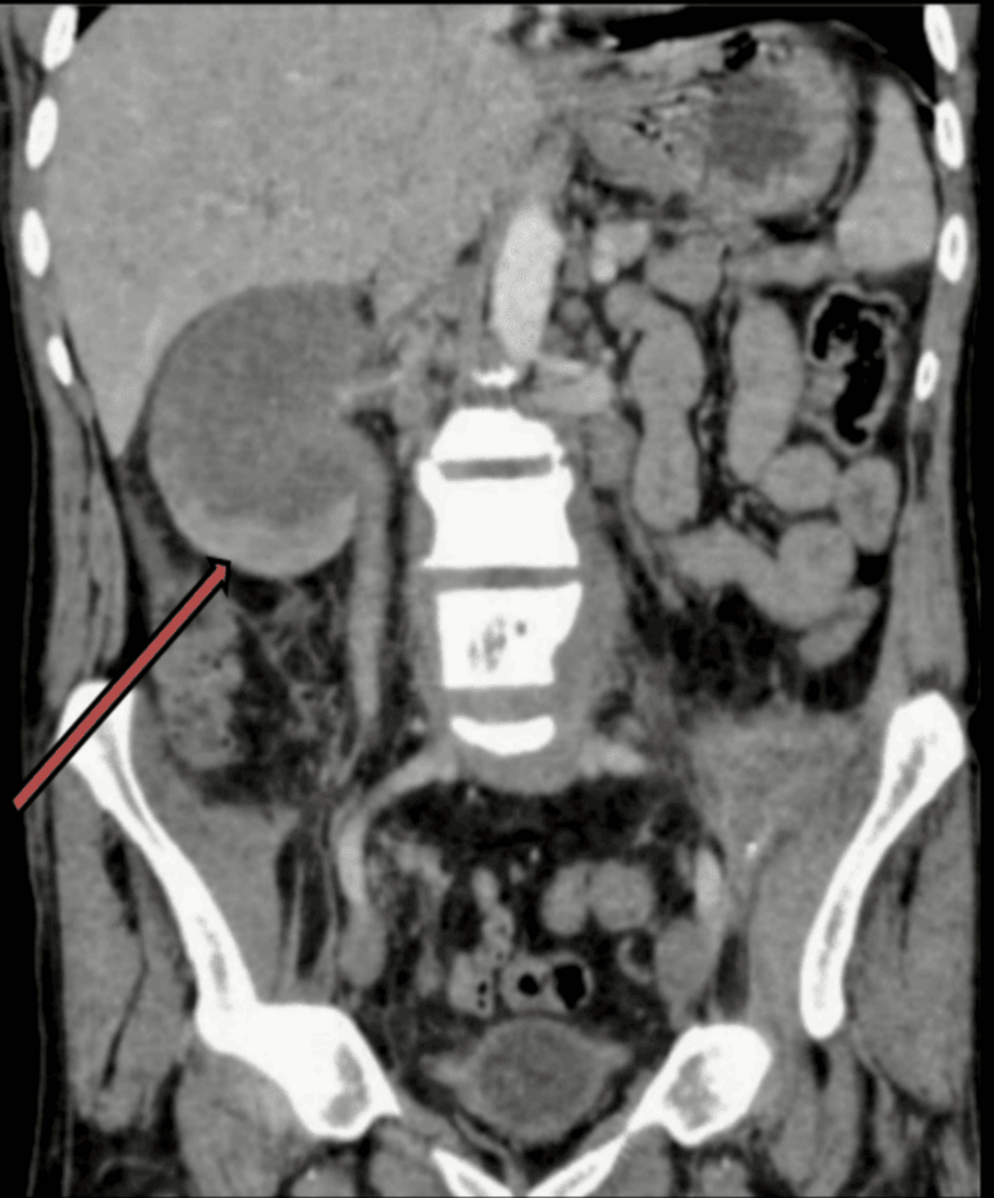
The coronal post-contrast CT image highlights the extent of the mass in the right kidney, emphasizing its peripheral enhancement. This view demonstrates the replacement of normal renal parenchyma in the hilar and perihilar regions by the tumor. The peripheral enhancement and central hypoenhancement patterns further support the suspicion of a malignant process, such as collecting duct carcinoma.
